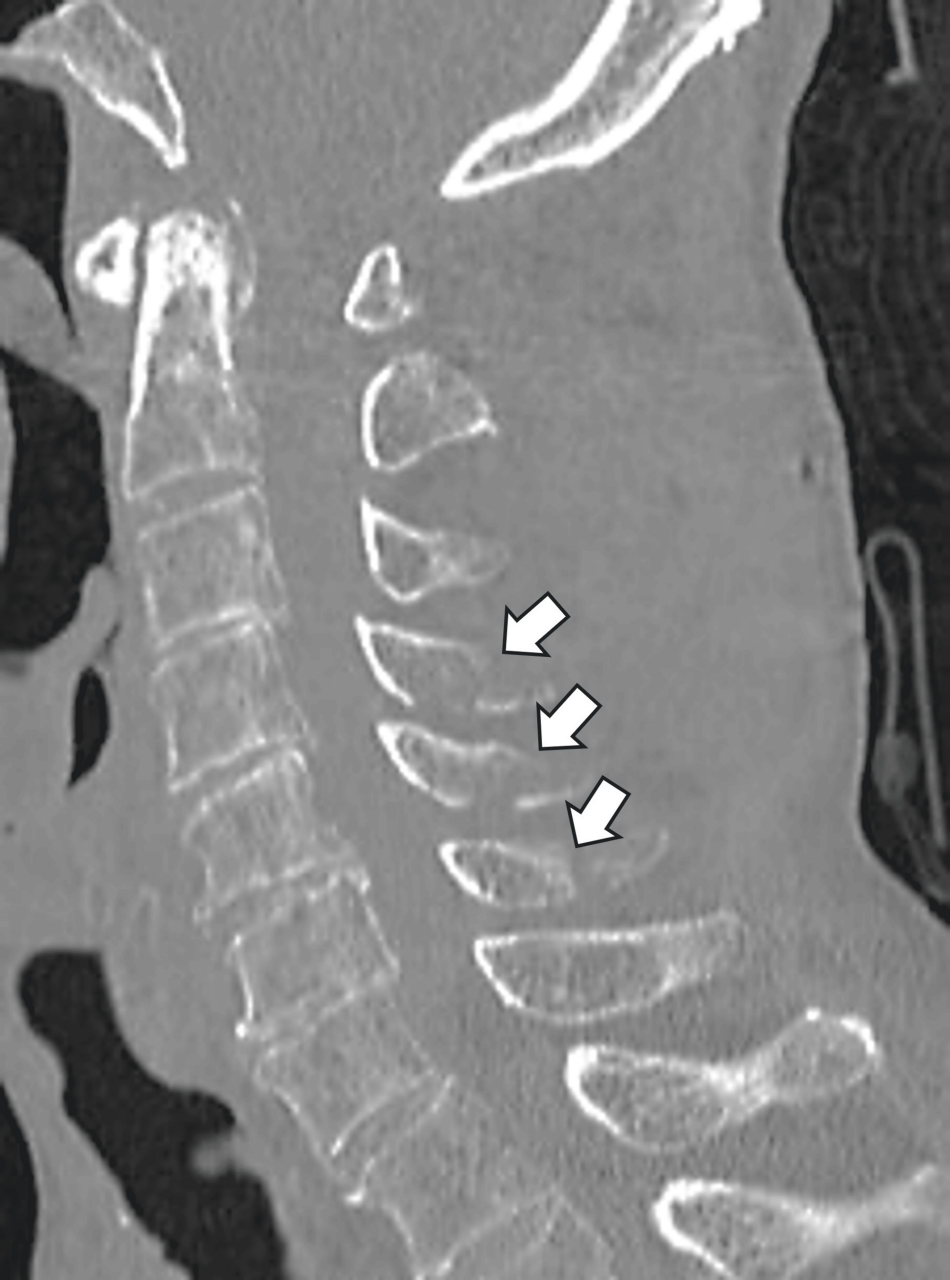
Sagittal reconstructed computed tomography (CT) image. Arrows point to tip fractures involving the superior and inferior articular processes at C4-C6.
